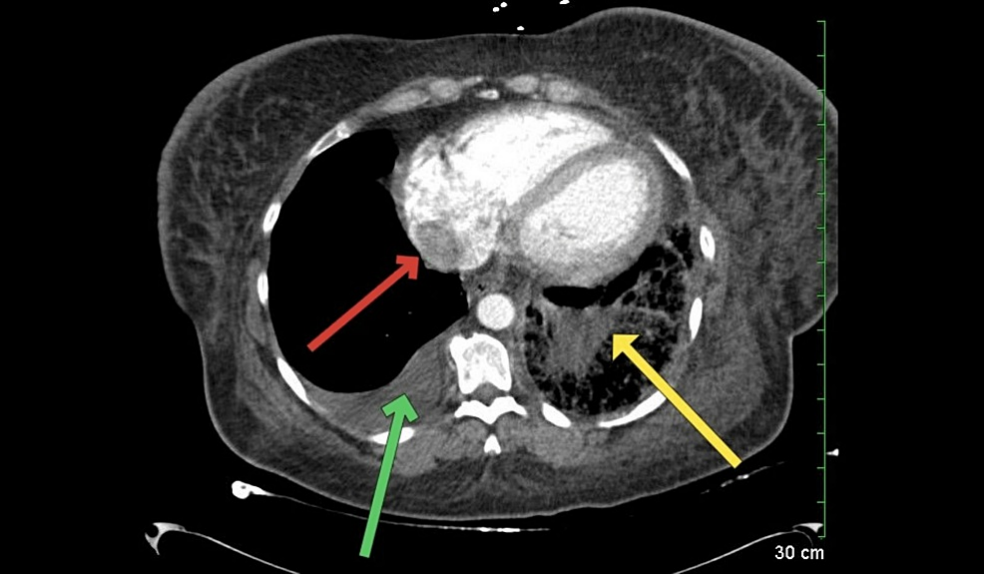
CT pulmonary angiography of the chest CT pulmonary angiography showing a red arrow pointing at the thrombus in the right atrium, a green arrow pointing at the right pleural effusion, and a yellow arrow pointing at a wedge-shaped consolidation in the territory of the pulmonary artery that was blocked by the pulmonary embolus CT: computed tomography
